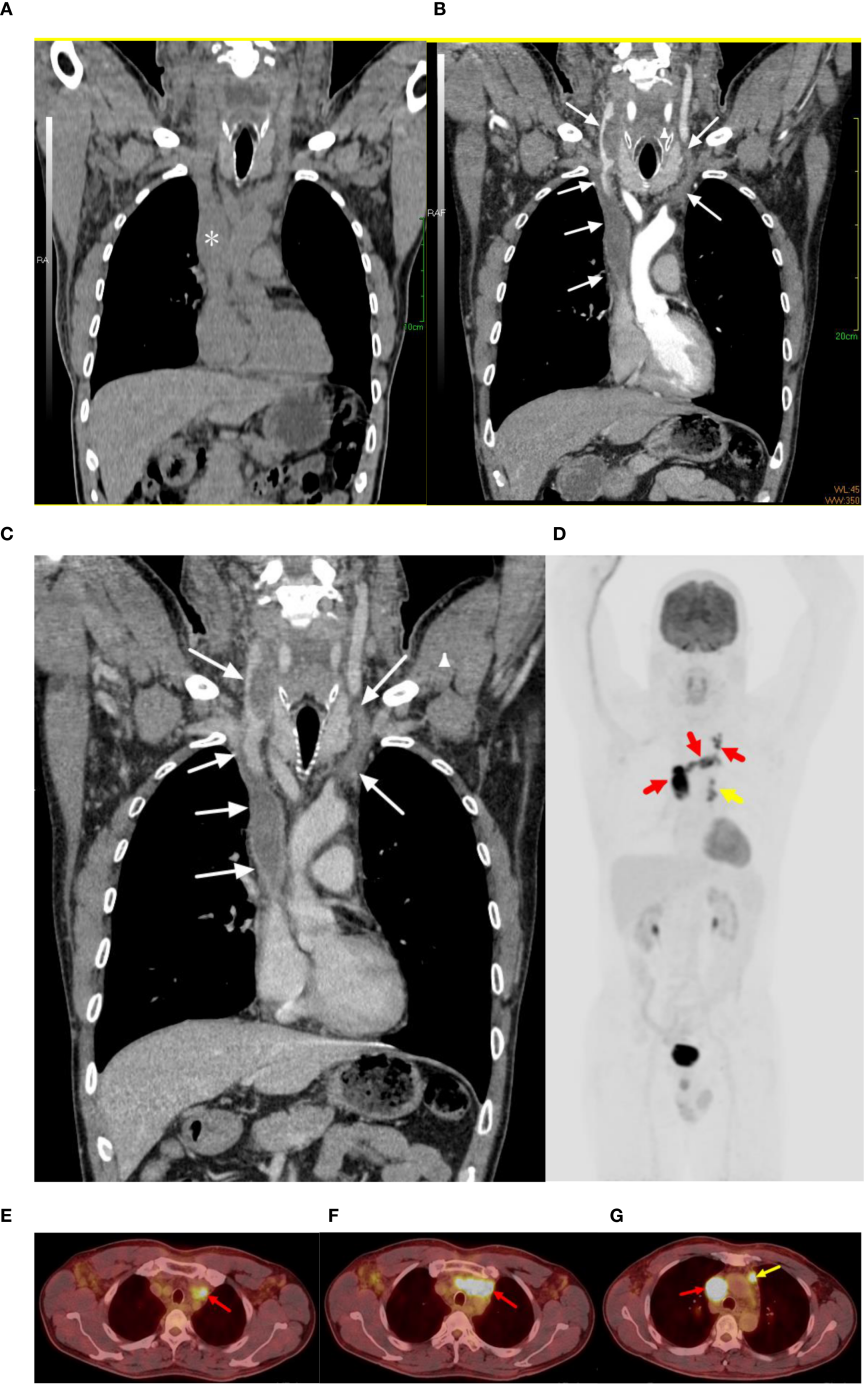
Contrast-enhanced CT and ^18^F-FDG PET images for the PISS patient. (A–C) Non-contrast CT reveals fusiform dilation of the superior vena cava. Contrast-enhanced CT revealed filling defects in the superior vena cava, brachiocephalic vein, and bilateral internal jugular vein (white arrows). (D–G)
